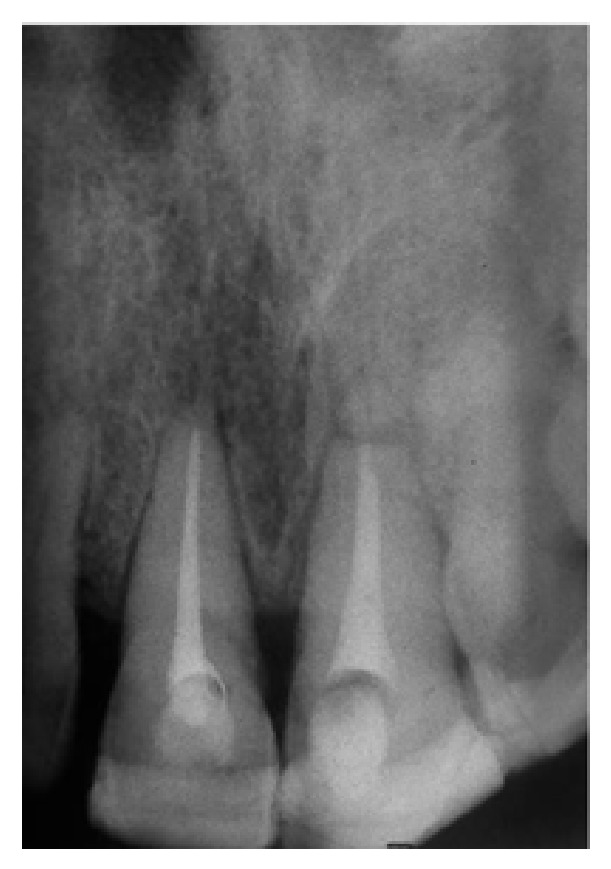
After 15-month radiographic control examination.
